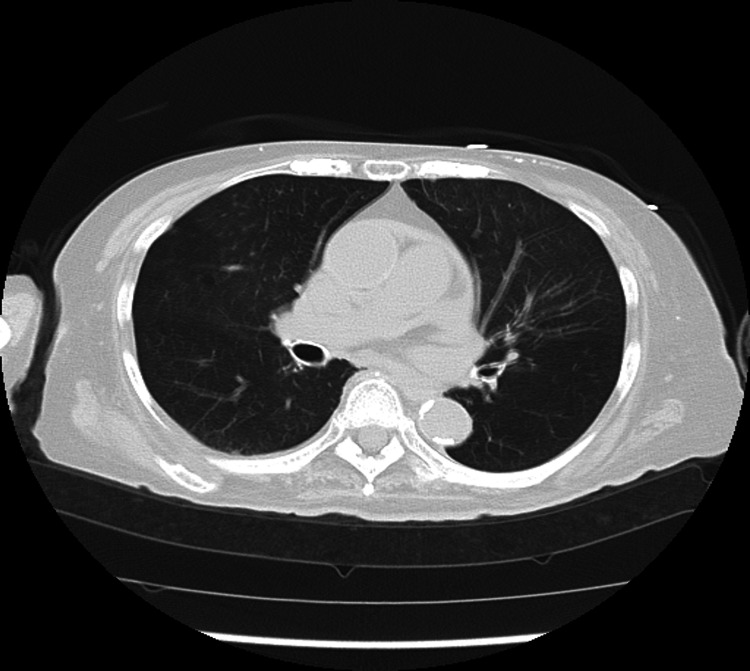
The lung field CT image of a COVID-19-positive patient (Case 1). There was no obvious image of pneumonia in the lung field.
